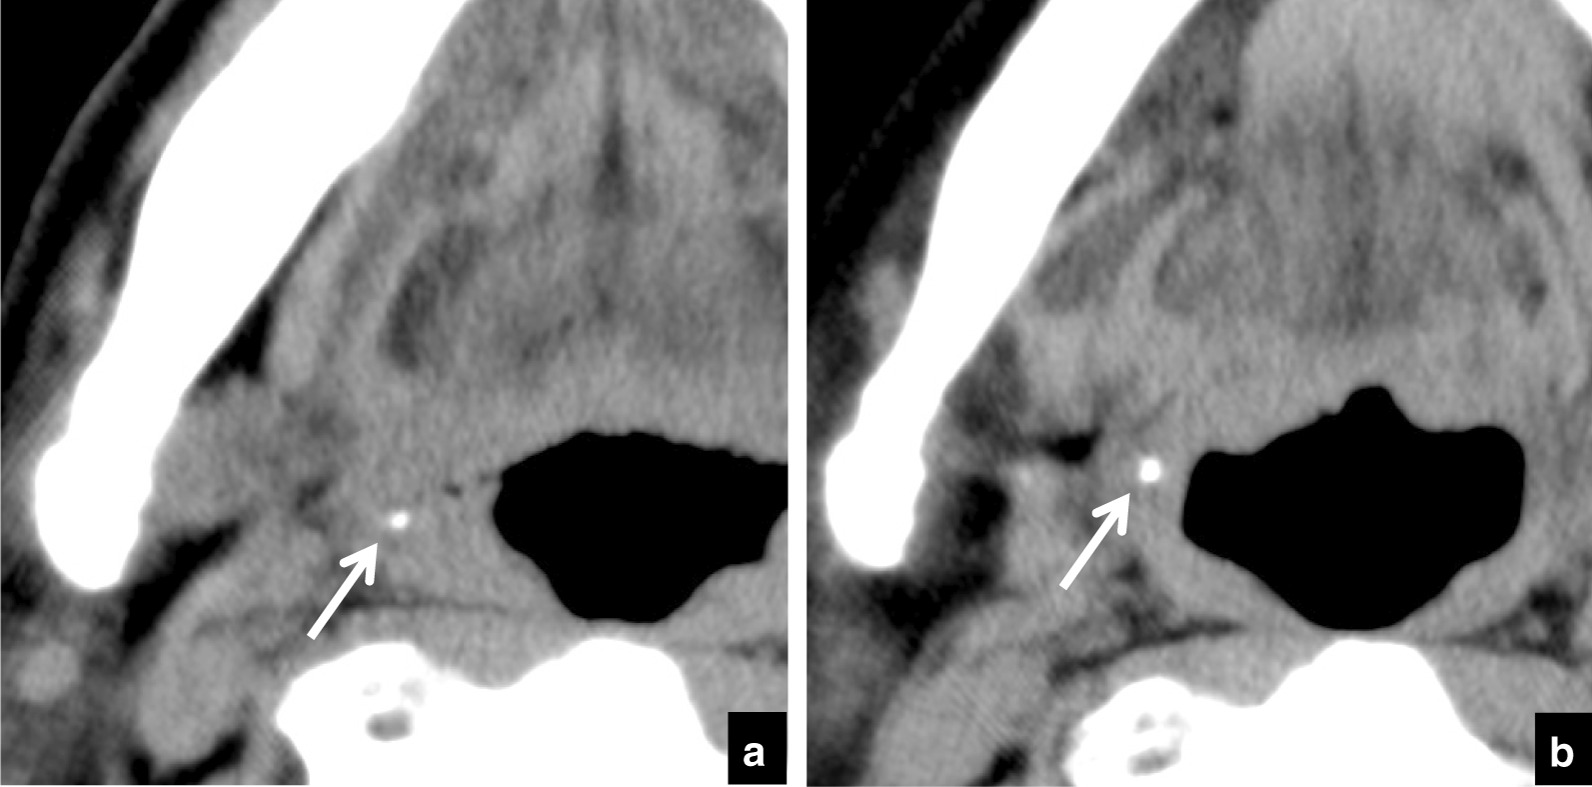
A 70-year-old subject with osteomyelitis of right mandibular gingiva in whom dynamic movement of the tonsillolith was seen toward the internal side on CT. a Axial CT image at the palatine tonsillar level at the initial examination. The depth of the tonsillolith (arrow) is 6.1 mm. b Follow-up CT image at the same level 48 months later. The depth of the tonsillolith (arrow) is now 3.3 mm, following movement in the medial direction (toward the respiratory tract)
